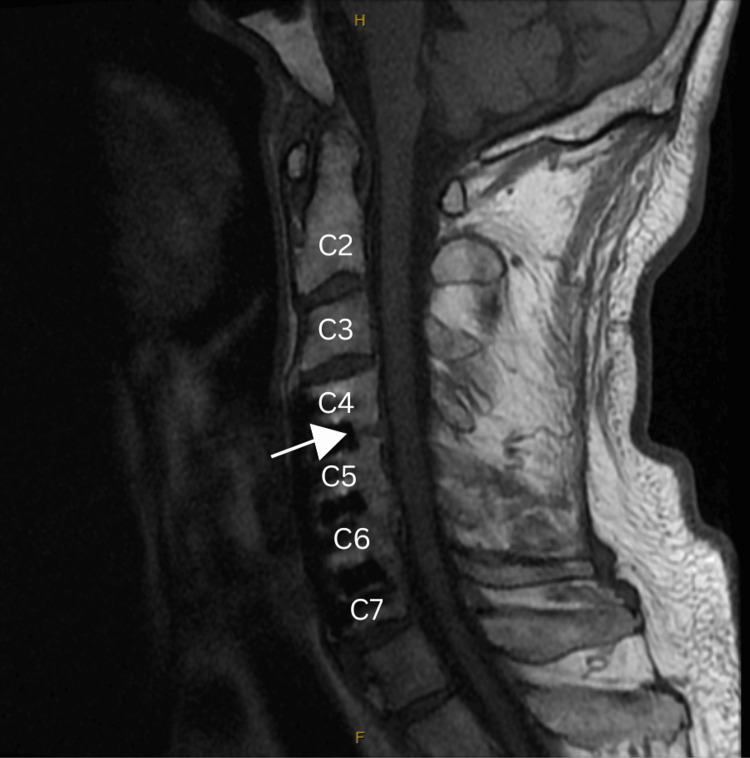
Cervical spine MRI Sagittal T1 MRI of the cervical spine demonstrating moderate to severe neural foraminal stenosis present at C4-C5 as indicated by the white arrow.
